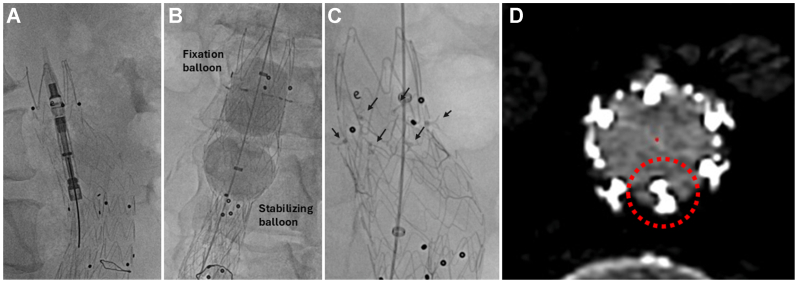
(A) Still image demonstrating unsheathing of the Aortoseal staple delivery system. (B) Dual-balloon catheter inflated within the endograft, with the stabilizing balloon positioned just above the endograft bifurcation and the fixation balloon within the Aortoseal scaffold. (C) Completion angiographic image after endostaple deployment. *Black arrows* demonstrate the location of the endostaples. (D) Cone-beam computed tomography (CT) image demonstrating circumferential distribution of endostaples along the nitinol frame. The *red dashed circle* indicates the nondeployed endostaple.
